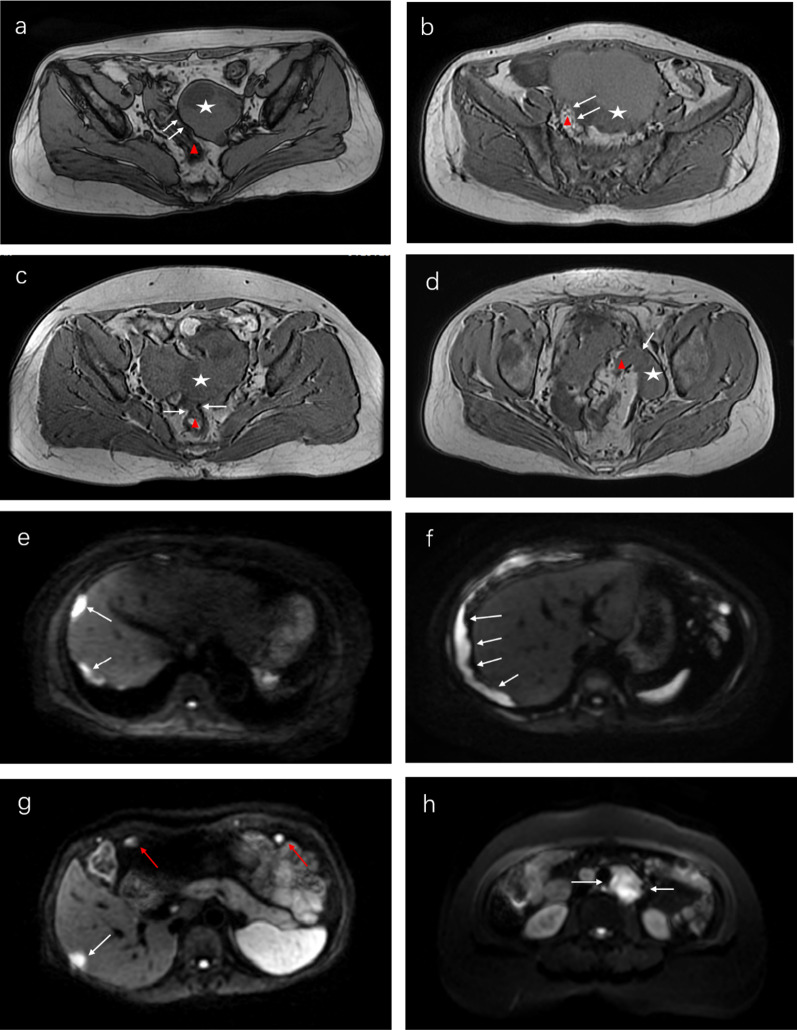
Image features of the MRI variables. a–d Four grades of relationship between the sigmoid colon/rectum and ovarian mass. a Axial MR T1 dual-echo (MR-T1-DE) image shows grade 0 (clear). A hook edge sign existed (white arrowheads) between the sigmoid colon (red triangle) and ovarian mass (white star). b Axial MR-T1-DE image shows grade 1 (close). The hook edge disappeared, but the shape of the sigmoid colon (red triangle) and ovarian mass (white star) can be vaguely distinguished (white arrowheads). c Axial MR-T1-DE image shows grade 2 (bridge sign). The hook edge disappeared, and the rectum (red triangle) and ovarian mass (white star) were limited adhered (white arrowheads). d Axial MR-T1-DE image shows grade 3 (fusion). The hook edge disappeared, and the sigmoid colon (red triangle) and ovarian mass (white star) fused into a block (white arrowheads). e, f Diaphragmatic metastasis. e Diffusion-weighted image shows hyperintense nodules implanted under the diaphragm (white arrowheads). f DW image shows extensive thickening of the diaphragm with hyperintensity (white arrowheads). g Metastases of liver and omentum. DW image shows hyperintense nodules of liver (white arrowhead) and omentum (red arrowheads). h Retroperitoneal lymphadenopathy. DW image shows significant hyperintensity of retroperitoneal enlarged lymph nodes (white arrowheads)
